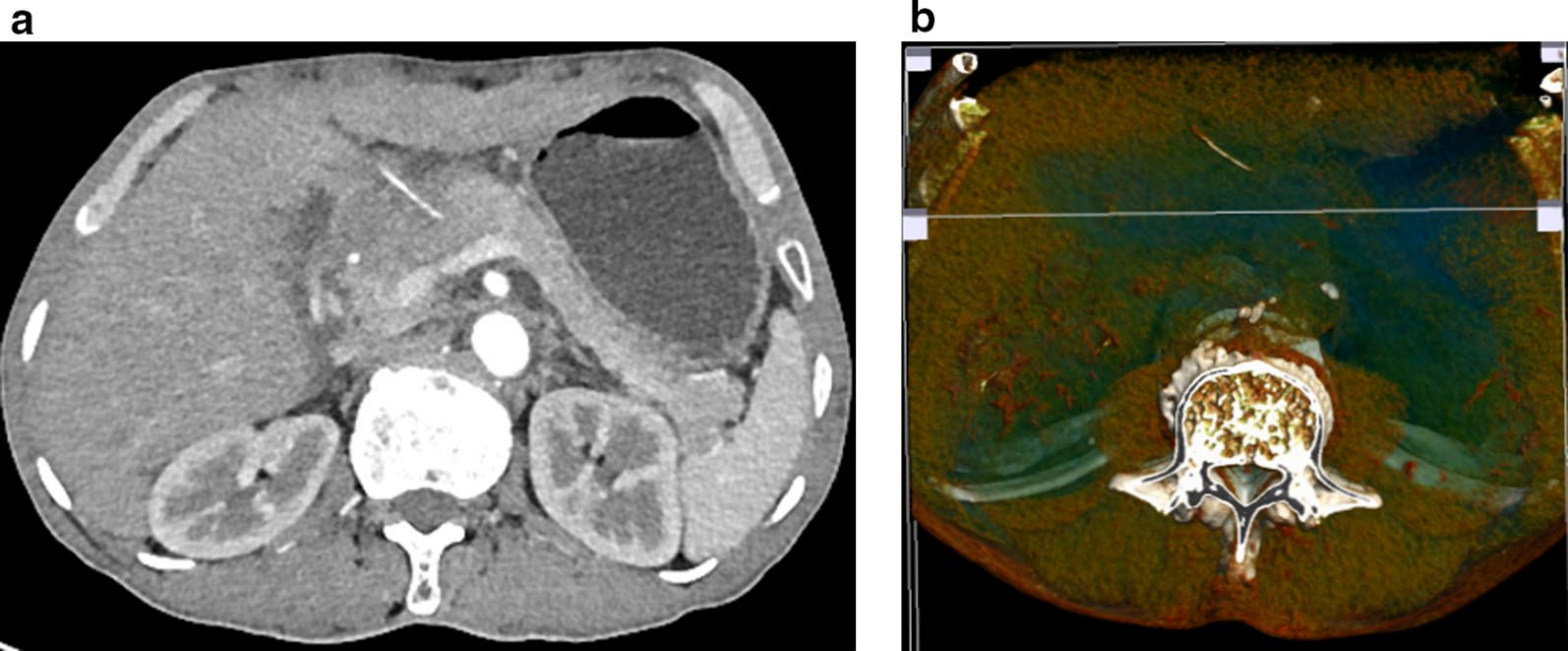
a Computed tomography scan of the abdomen revealed a linear, hyperdense, foreign body along the stomach wall and pancreatic neck. b The bone condition CT clearly shows the position and shape of the fish bone in the abdominal cavity
